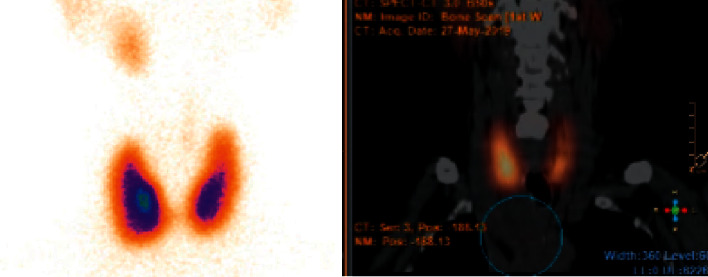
^99m^Pertechnetate scintigraphy showing increased diffuse uptake in the thyroid parenchyma and the absence of uptake in the mediastinal mass.
